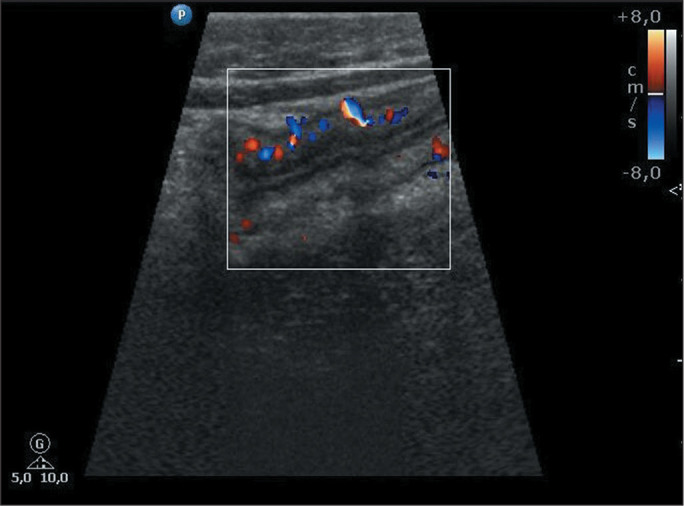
Color Doppler ultrasound image of a 13-year-old male patient with Crohn’s
